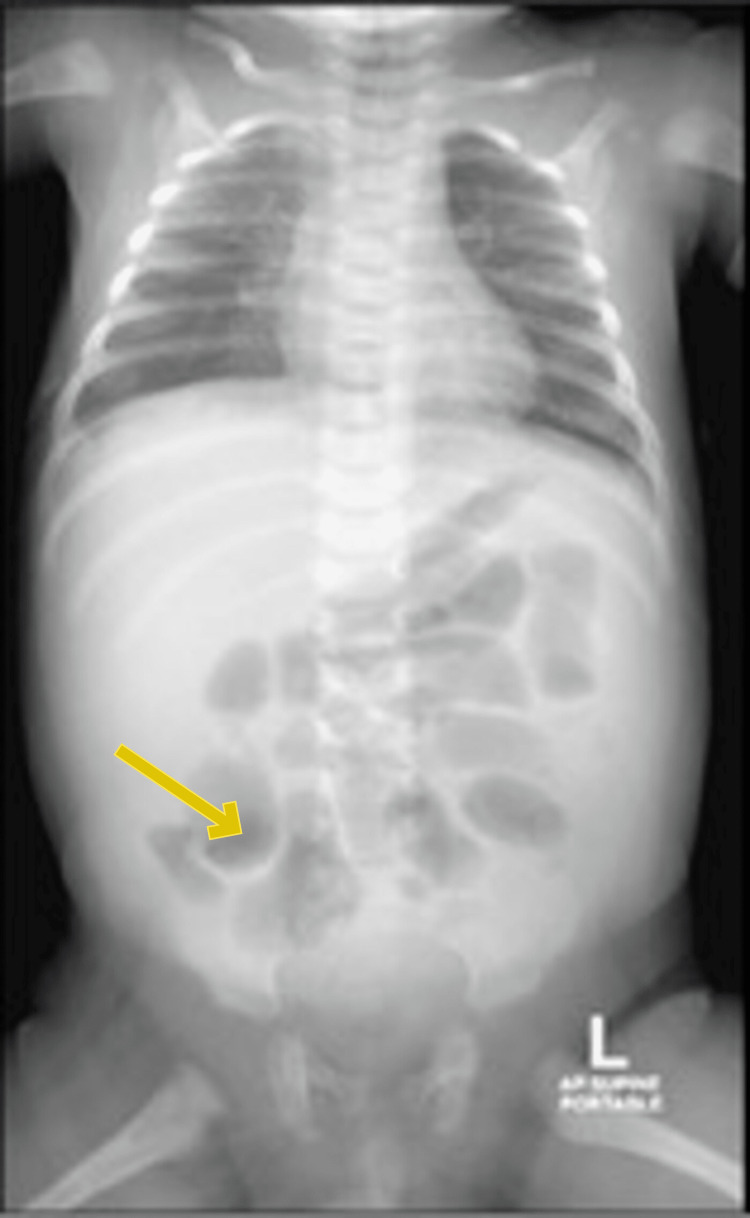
X-ray showing increased bronchovascular markings, and gut gases
